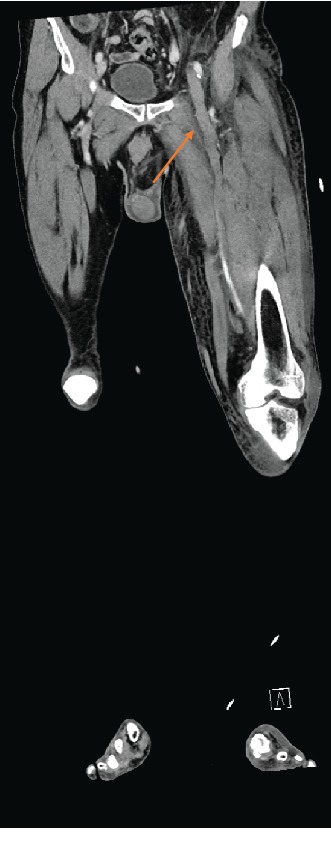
Angio-CT of the patient showing complete thrombosis of the left femoral vein (arrow).
